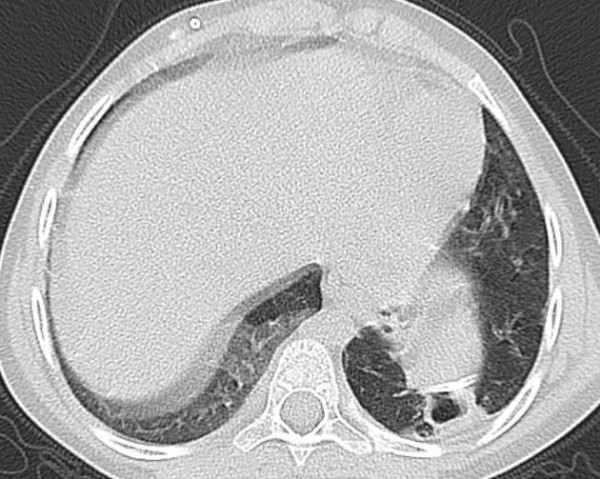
In computed tomography of thorax, the cavitary lesion with a diameter of 2 cm at lower lobe of left lung, and the catheter end at left hemithorax.
